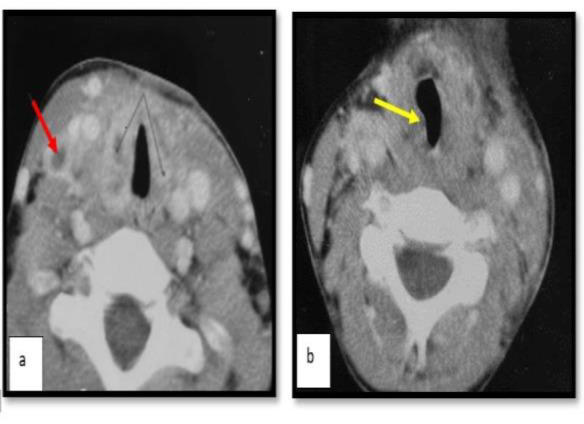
Cervical and thoracic CT scan: suspicious enlargement of the thyroid gland associated with cervical adenopathy and suspicion of laryngeal extension.
